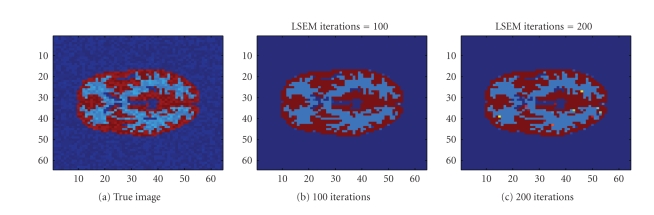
64 × 64 segmented MRI slice of the brain, nonpiecewise constant by adding Rand() functions.
